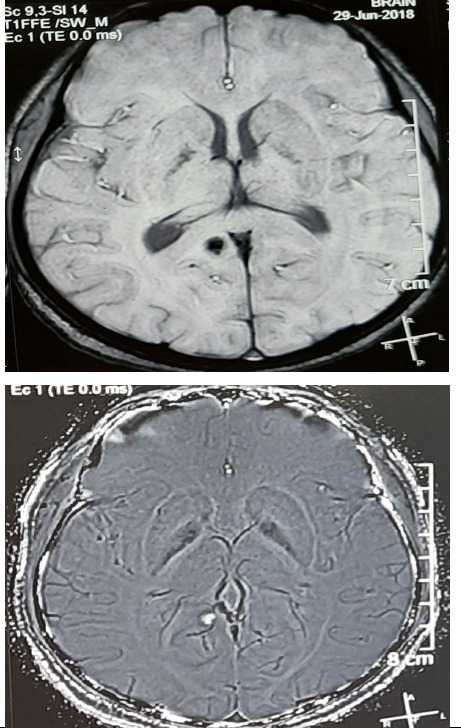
MRI shows calcification in the brain.
